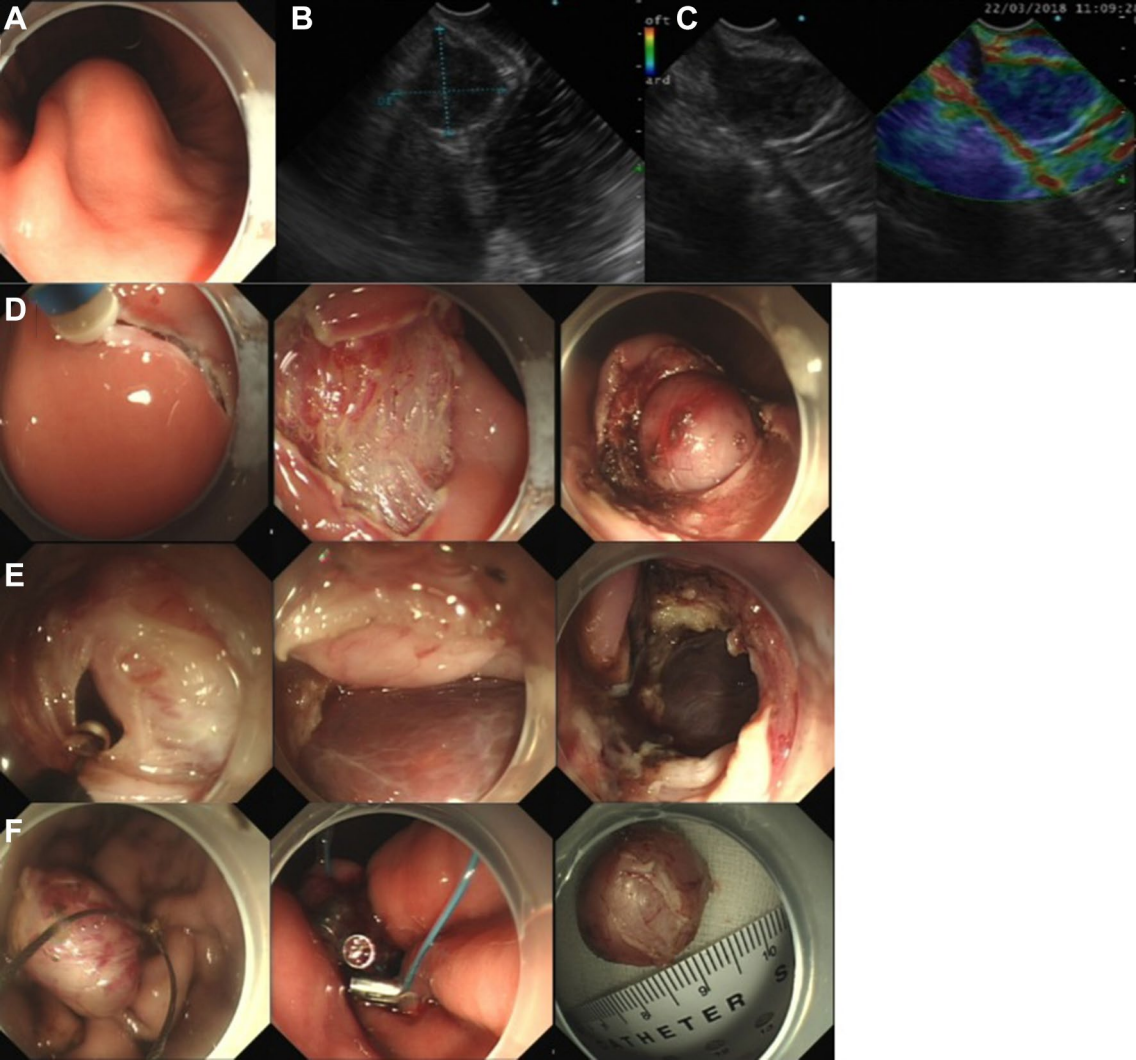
Separate maneuvers of EFTR for a muscularis propria (MP)-originating gastric submucosal tumor (G-SMT): A the protrusive submucosal lesion with smooth surface at the gastric greater curvature, B, C this well-demarcated and heterogeneously hypoechoic lesion showing moderate enhancement on the contrast-enhanced endoscopic ultrasound (EUS), D circumferential pre-cutting around the lesion and separating the submucosal tissue to expose the tumor, E separating the muscularis propria tissue associated with the tumor and creating an active perforation, F completely snaring the target tumor, closing the gastric wall defect with purse-string closure method by nylon cord and multiple metallic clips
